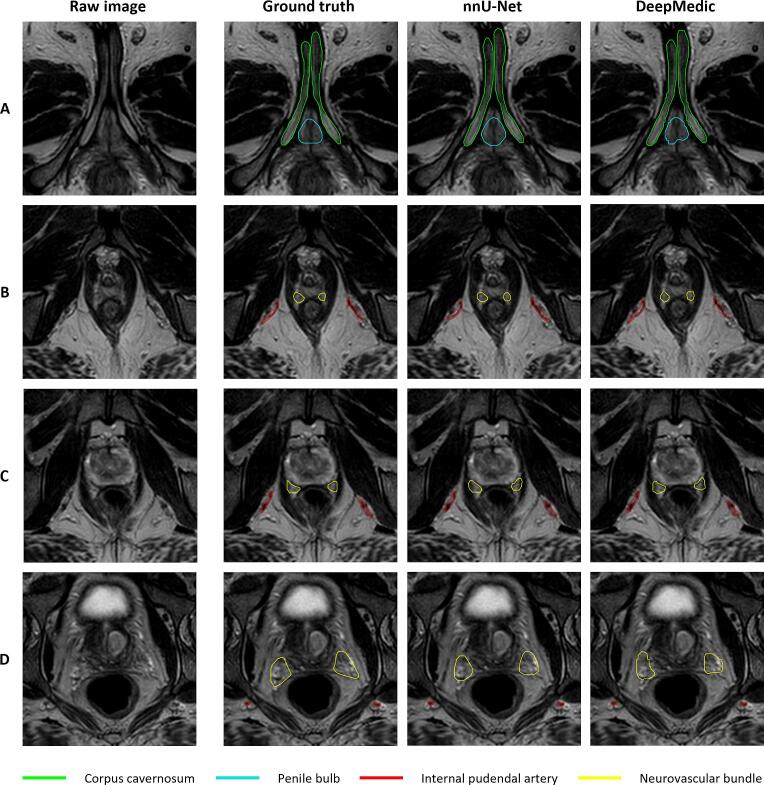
Representative case with the penile bulb (cyan), corpus cavernosum (green), internal pudendal artery (red), and neurovascular bundle (yellow) contours on T2-weighted MRI. Axial MR images obtained at the level of the penile bulb and corpora cavernosa (A), prostate apex level (B), prostate midgland level (C), and prostate base level (D). (For interpretation of the references to colour in this figure legend, the reader is referred to the web version of this article.)
